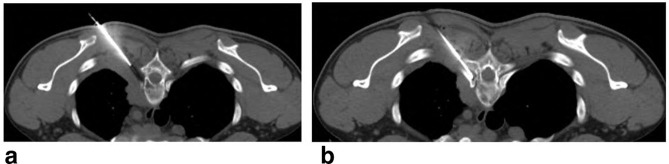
CT guidance biopsy left paraspinal mass.
